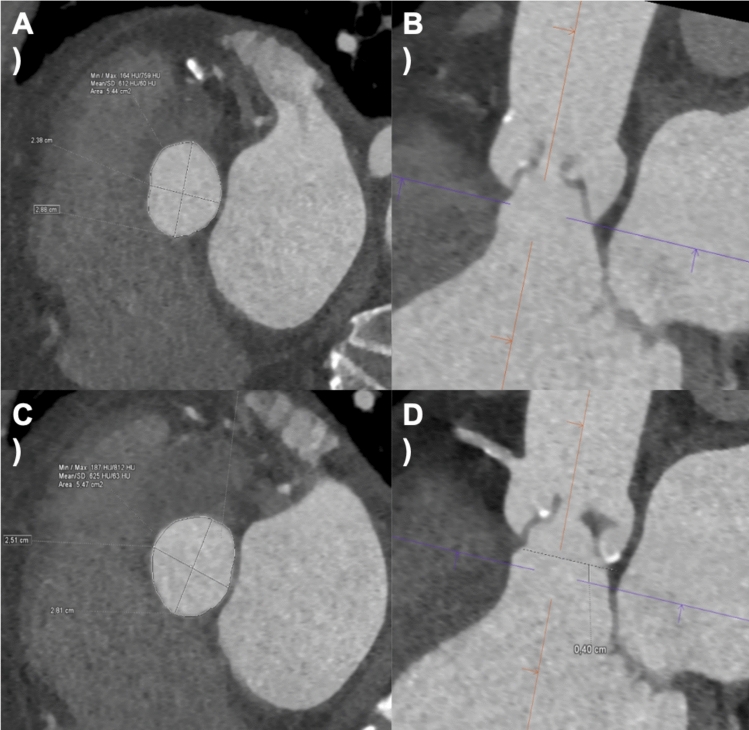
Pre-TAVI-CTA measurements. The figure shows the CTA measurement of aortic annulus area (A, B minimal and maximal diameter) and measurement of LVOT area (C, D minimal and maximal diameter) 4 mm below the aortic annulus. *CTA* computed tomography angiography, *LVOT* left ventricular outflow tract, *TAVI* trans-catheter aortic valve implantation
